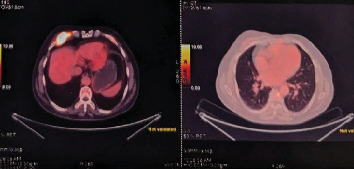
PET CT postchemotherapy showing the chest wall invasion.
